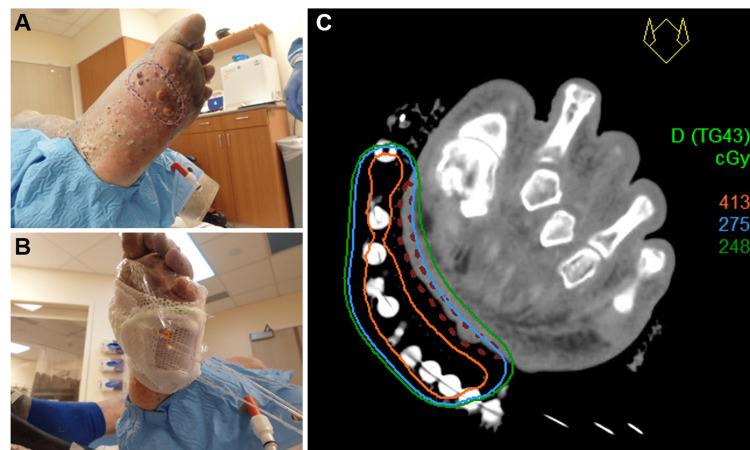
(A) Marker wire surrounding the left sole lesions; (B) Aquaplast mold applicator; (C) a single fractional isodose line distribution on an axial view of the CT planning dataset for the 27.5 Gy in 10 fractions prescription (Rx) for the drawn CTV (dark red dashed lines). Isodose lines for fractional doses of 275 cGy (Rx), 248 cGy (90% of Rx), and 413 cGy (150% of Rx) are shown in blue, green, and orange, respectively. cGy: centigray; CTV: clinical target volume
